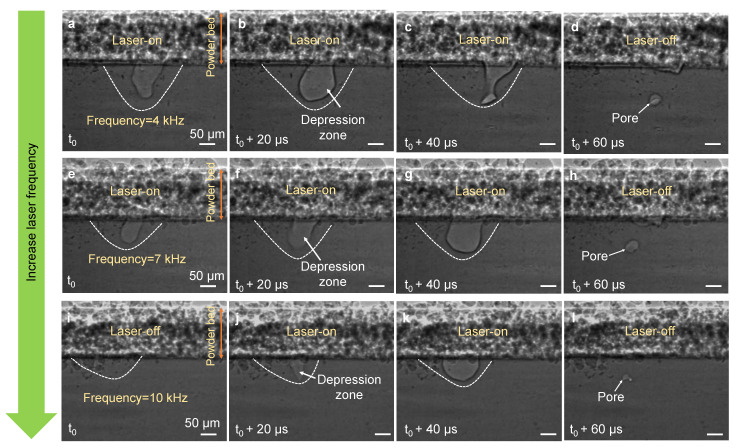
Pore formation under varying laser frequencies at a constant laser duty cycle: (a–l) Dynamic X-ray images showing pore formation during the PW-LPBF of Al6061 at laser frequencies of 4 kHz (a–d), 7 kHz (e–h), and 10 kHz (i–l) under a laser duty cycle of 50%, a laser power of 470 W and a scan speed of 0.4 m/s. In d, h and l, the melt pool boundary is not indicated to avoid blocking the view of the pores. Note that images do not display the complete duration of one pulse. The compiled movies showing the complete duration of two consecutive pulses are available in the Supplementary Material (Movies S1–S3).
